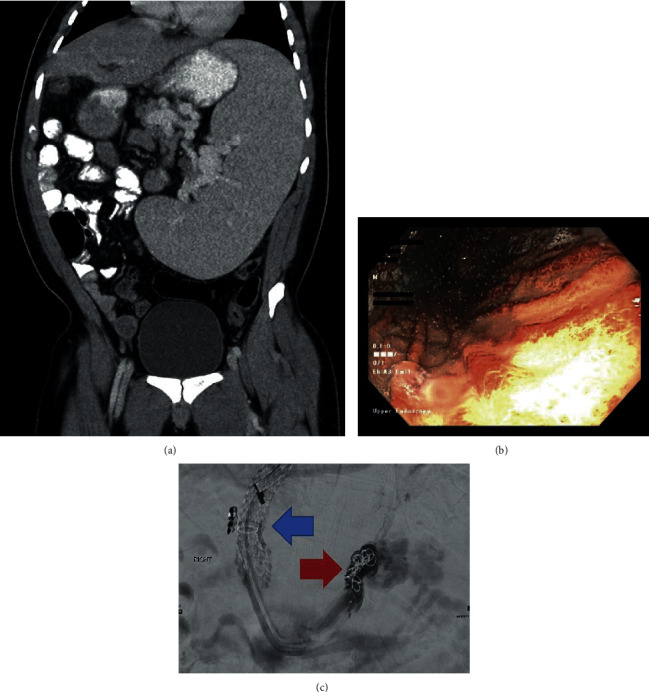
(a) Non-contrast-enhanced CT scan of the abdomen and pelvis showing splenomegaly with a craniocaudal length of 23 cm and portosystemic collaterals. (b) Endoscopic image of the stomach showing diffuse portal gastropathy. (c) Fluoroscopic image following TIPS placement (blue arrow) and coiling of the bleeding esophageal varix (red arrow).
